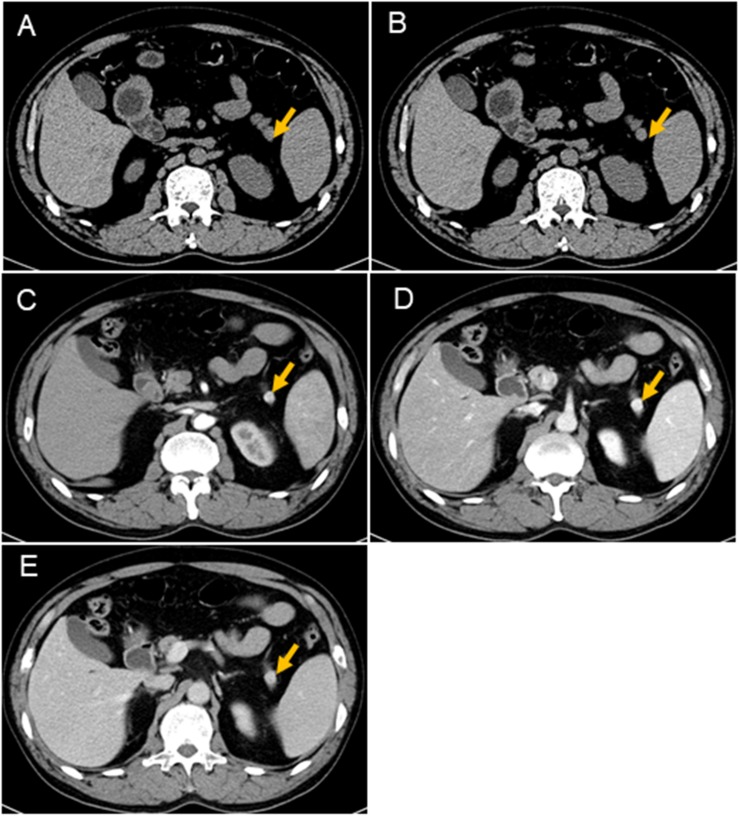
CT scans of the pancreas. Nodules (arrows) can be seen in the tail of the pancreas. It is not on the same level as the pancreas, which results show a lack of the same layer contrast. The CT values of the nodules are similar to the CT values of the pancreas (A,B). The size of the mass is about 1.1 cm × 1.2 cm, and the boundary is clear. The mass is significantly enhanced at the arterial phase, and the CT value is about 125HU (C); the parenchymal phase is significantly enhanced, and the CT value is about 170HU (D); the venous phase is significantly enhanced, and CT value About 164 HU (E).
